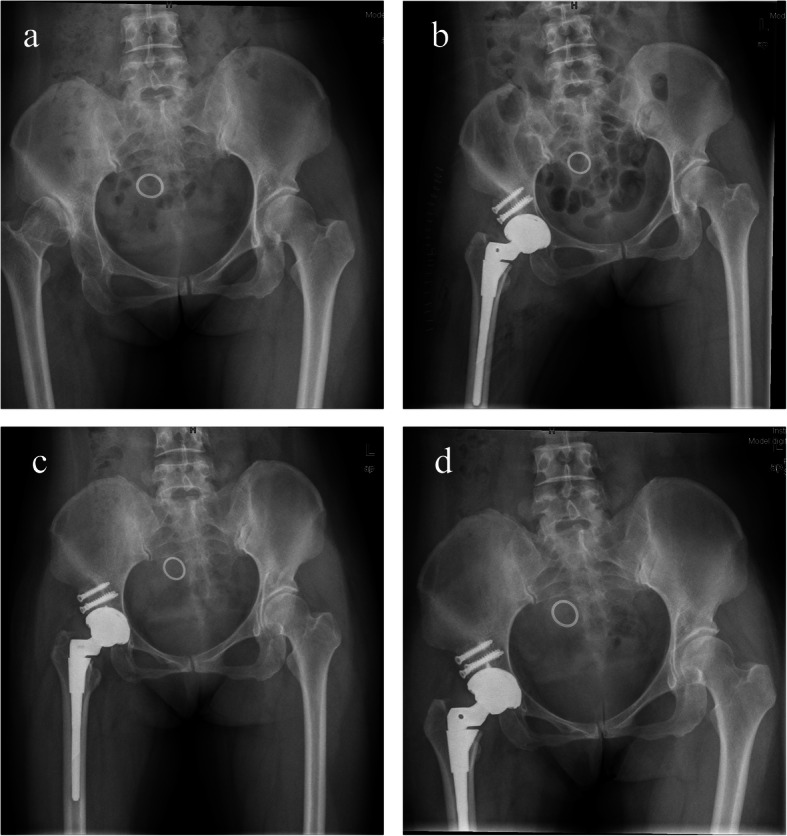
Case presentation of structural autograft with a bulk femoral head for THA. a A 51-year-old woman was diagnosed with DDH Crowe III on the radiograph of the pelvis preoperatively. b The radiograph of the pelvis after surgery immediately showed the rate of cup coverage was 95% and the leg-length discrepancy was 0.16 cm. c The radiograph of the pelvis at 1-year follow-up showed the interface between the graft and the host bone had been obscure and no aseptic loosening of the component. d The radiograph of the pelvis at 84-month follow-up showed incorporation of grafted bone and no aseptic loosening and migration of the component
